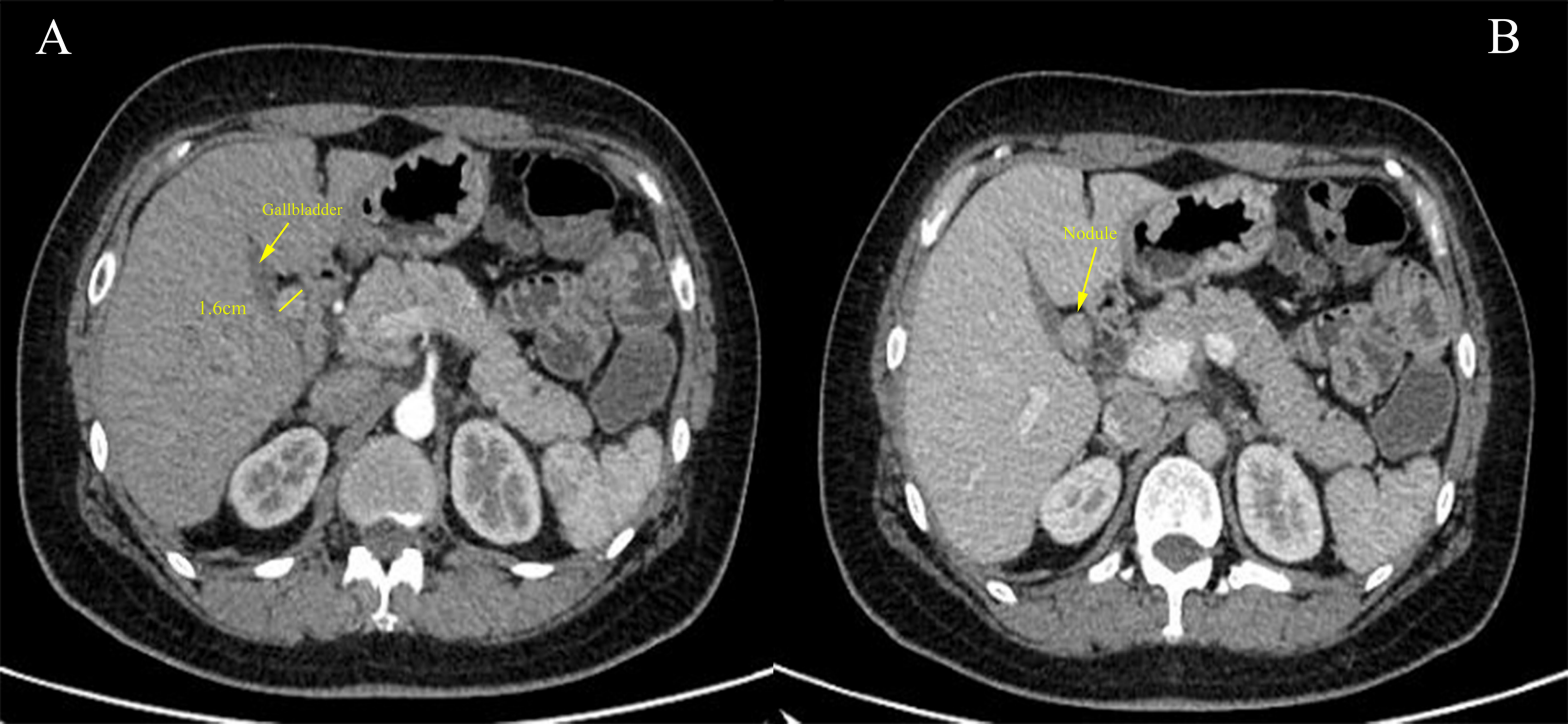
(A) Cross-sectional image of the contrast-enhanced abdominal computed tomography (CT) scan demonstrated a 1.6-cm nodule (yellow solid line) adjacent to the gallbladder with significant enhancement during the arterial phase. (B) The nodule showed continuous enhancement in the portal phase.
